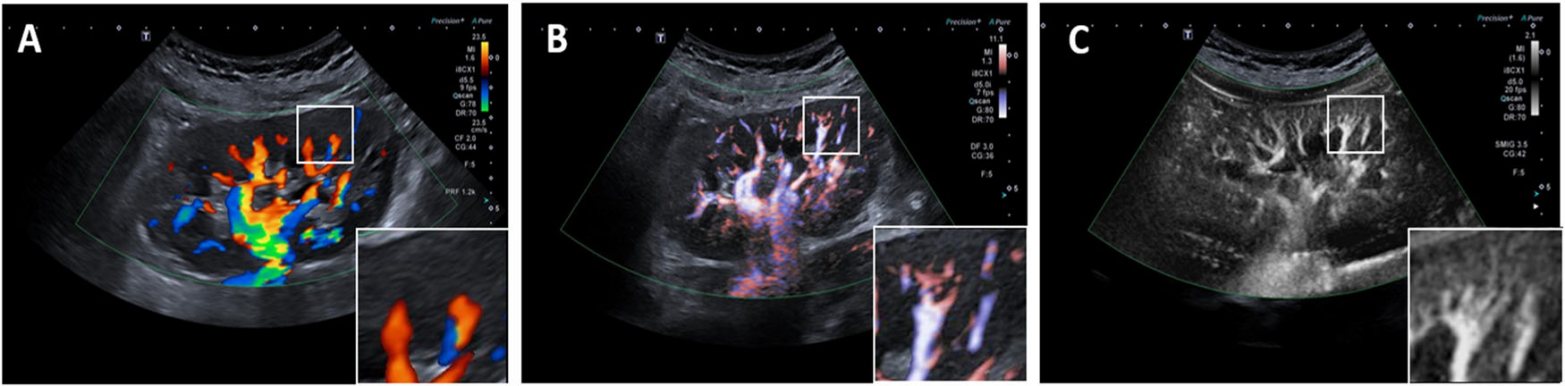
Evolution of Doppler mode in detection of smaller vascular beds. A Colour Doppler, (B) advanced dynamic flow, (C) SMI imaging. Insets: Cortex area and degree of measured vasculature with flow.
